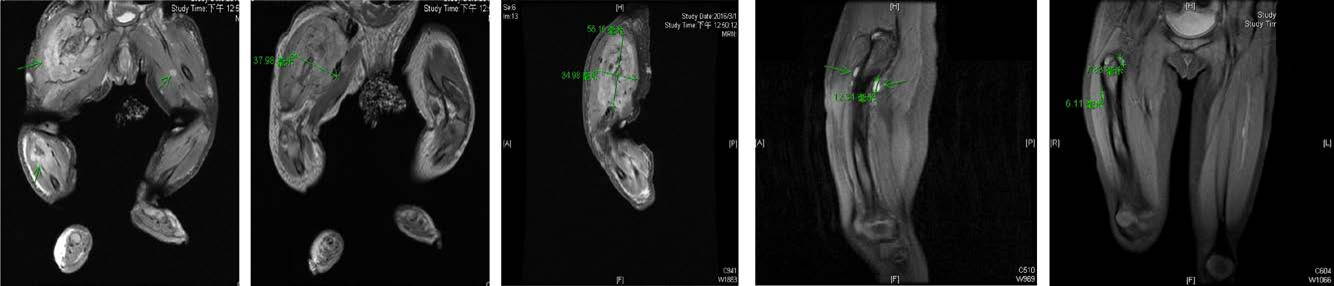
Musculoskeletal MRI showed multiple skin and soft tissue nodules throughout the lower trunk and both lower extremities with bone involvement. MRI = magnetic resonance imaging.
